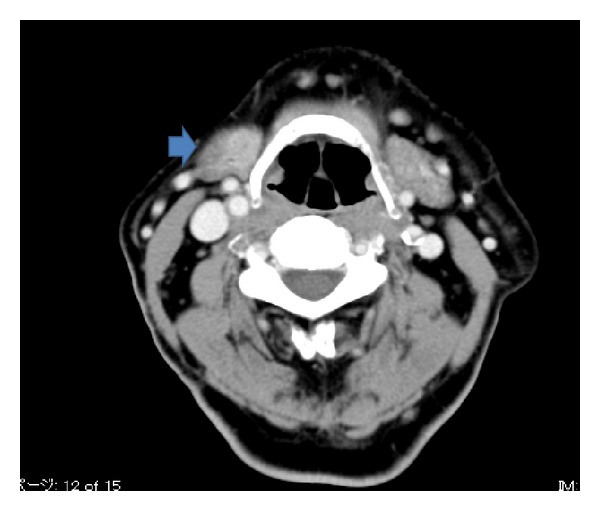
Neck CT showing a 20 mm × 10 mm nodular shadow with slight enhancement in the right submandibular area.
